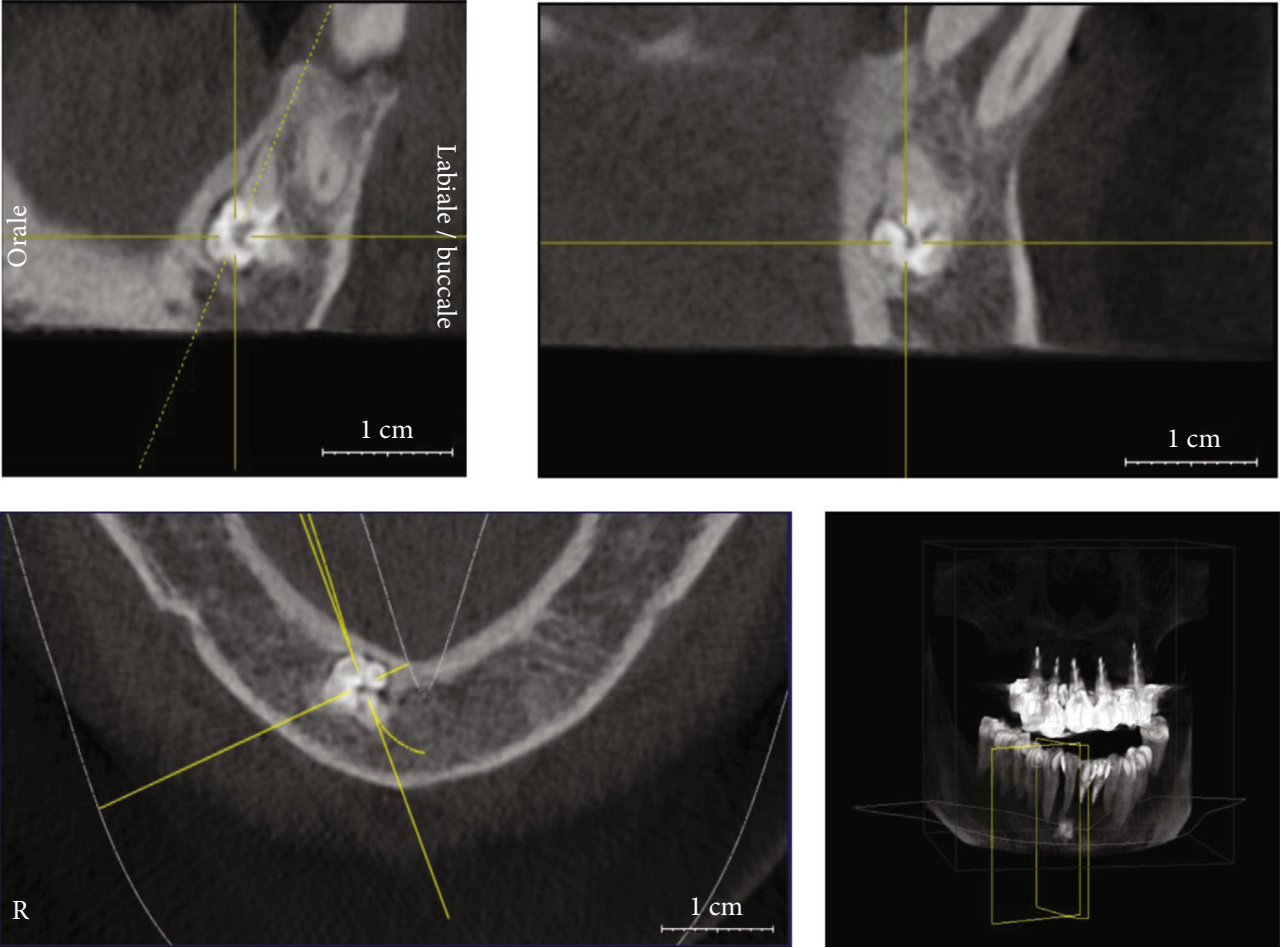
The image shows the size of the lesion in the CBCT scan approximately 10 years before its removal.
